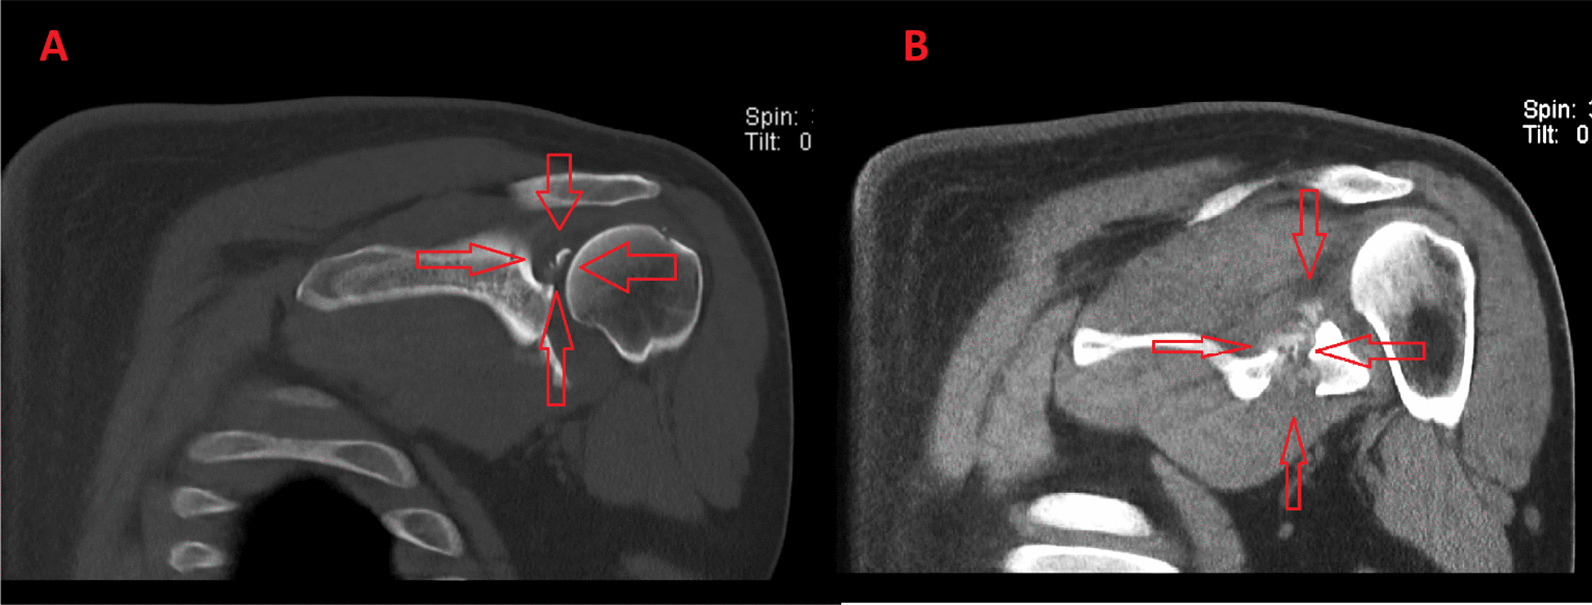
Coronal views of the shoulder CT scan. The red arrows indicate comminution in the region of the superior fracture line (A). The red arrows indicate callus formation at the fracture site (B)
